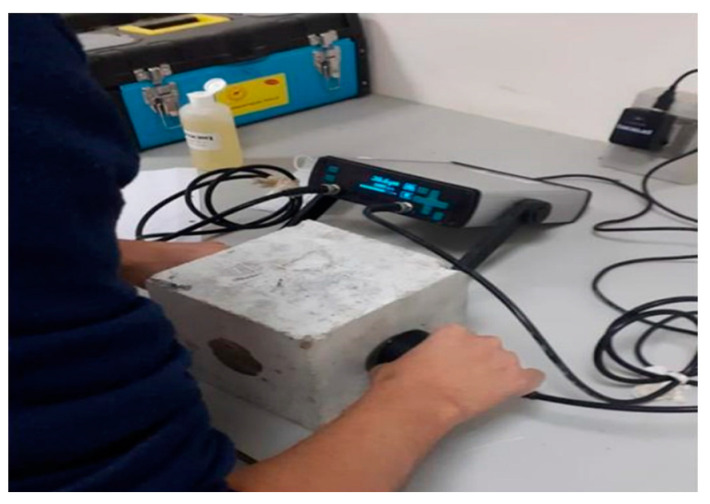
Ultrasonic pulse velocity test [45].
